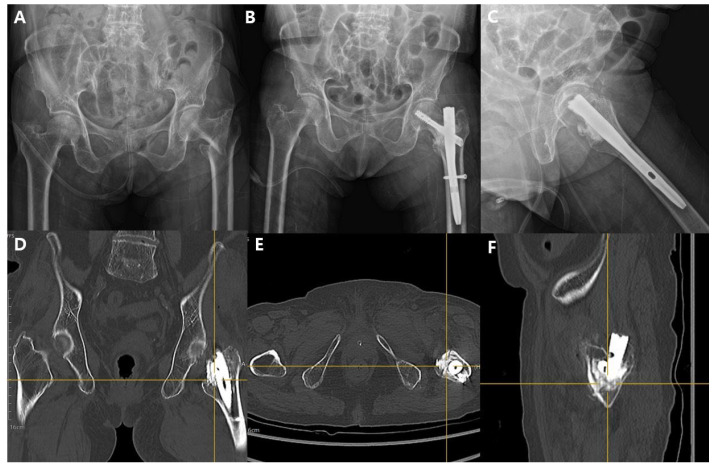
Pre- and postoperative imaging demonstrating cephalomedullary nailing with injectable calcium-based augmentation. (A) Preoperative anteroposterior (AP) pelvis radiograph showing an intertrochanteric fracture of the left femur. (B) Immediate postoperative AP pelvis view after cephalomedullary nailing. (C) Lateral postoperative view of the proximal femur; radiopaque material is visible along the head–neck track, consistent with ICCBS delivery via the fenestrated head element. (D–F) Multiplanar postoperative CT reconstructions—(D) coronal, (E) axial, and (F) sagittal planes—confirm circumferential, cloud-like distribution of the augmentation around the head element within the femoral head/neck, without intra-articular or extraosseous leakage (AP, anteroposterior; CT, computed tomography).
